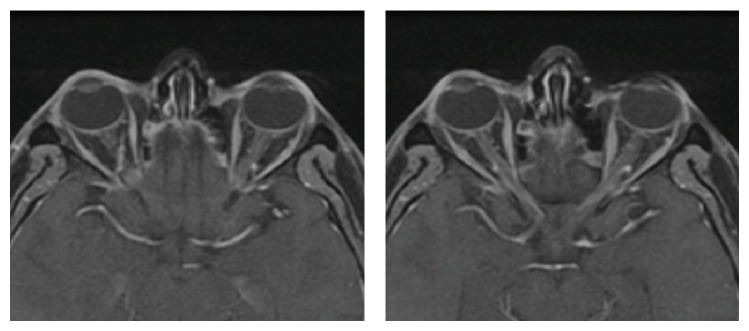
Optic MRI without abnormalities.
